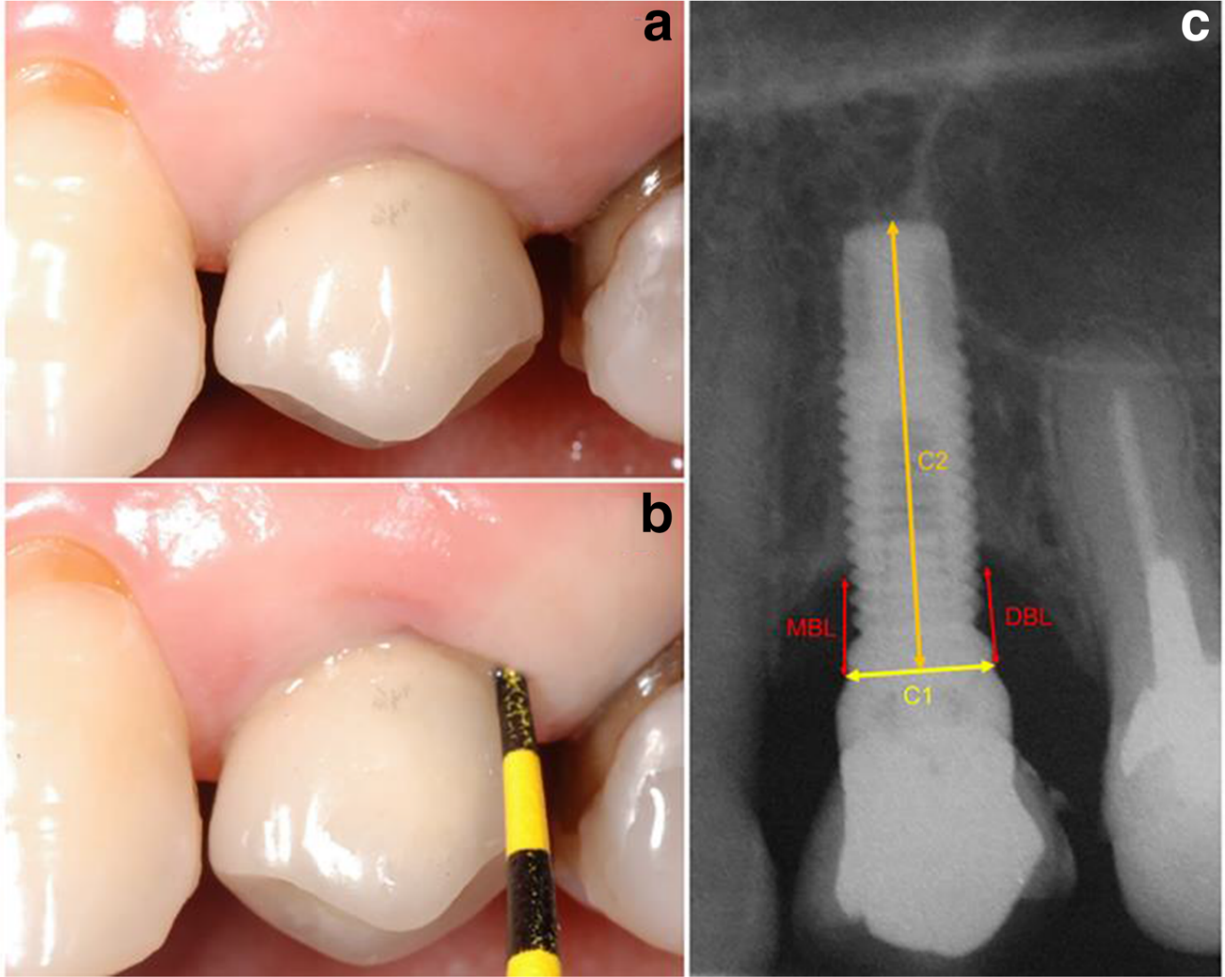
a Clinical view of an implant-supported single crown (ISSC). b Probing depth measured around an implant-supported single crown (ISSC). c Peri-implant bone loss was measured on both sides of each implant platform (DBL, distal bone loss; MBL, mesial bone loss) as the distance between the implant-abutment interface and the first bone-to-implant contact. To correct the dimensional distortion in the radiograph, the software was calibrated according to the true implant diameter (C1) and length (C2)
